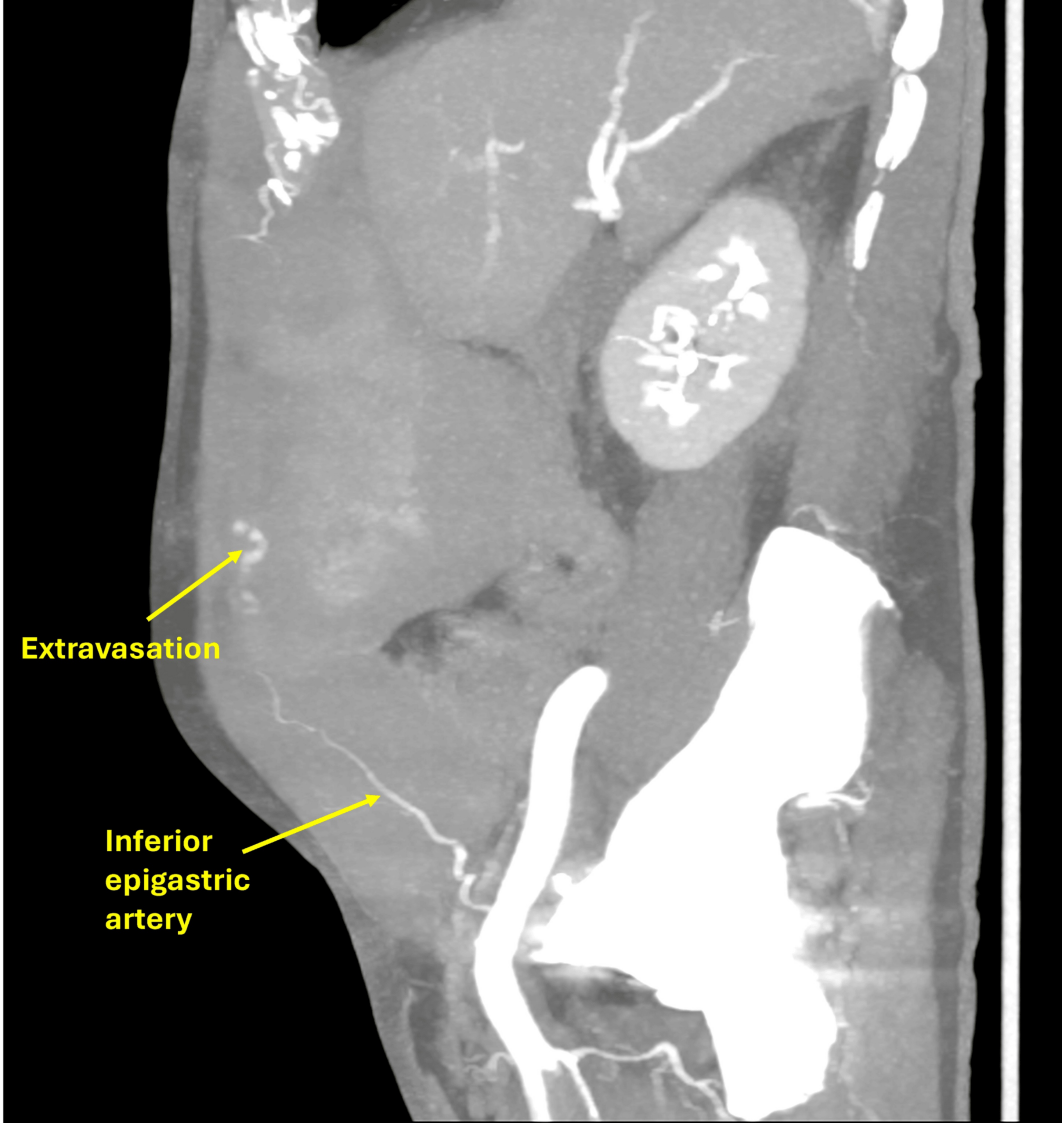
CT of the abdomen showing extravasation in the region of the right inferior epigastric artery.
